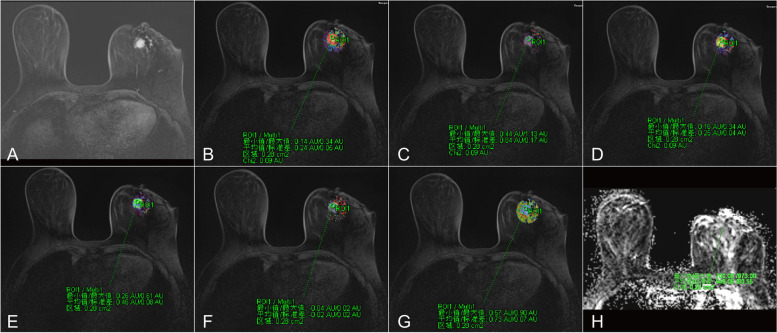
MR images of a 65-year-old woman with invasive ductal carcinoma of the left breast before NAC. A TWIST-DCE image. B–H Pseudocolor images for determining K^trans^ (B), K_ep_ (C), IAUC (D), W-in (E), W-out (F), TTP (G), and ADC (H). Red represents high values, yellow intermediate values, and blue low values. The values for K^trans^, K_ep_, IAUC, W-in, W-out, and TTP were 0.309 min^-1^, 1.136 min^-1^, 0.336, 0.499 min^-1^, 0.013 min^-1^, 0.582 minutes and 0.849×10^-3^ mm^2^/s, respectively
